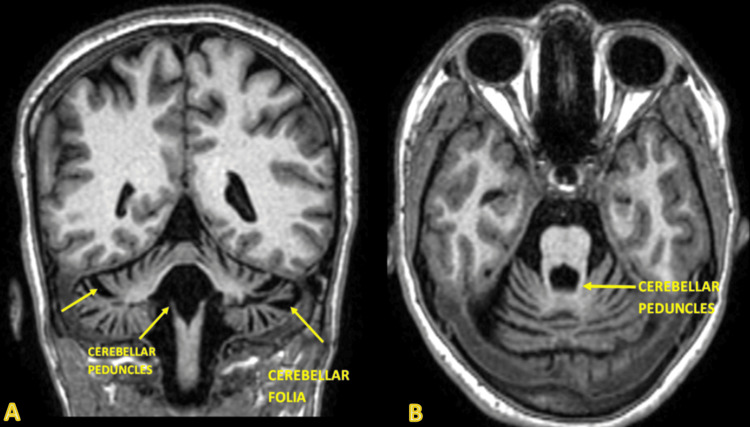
Coronal (A) and axial (B) T1-weighted image shows a front-to-back view of the brain. There is a clear atrophy of the cerebellar hemispheres with thinning of the cerebellar folia, cerebellar peduncles, and increased prominence of the cerebellar fissures. The fourth ventricle appears dilated. This image helps to assess the degree of cerebellar atrophy and its impact on surrounding structures.
